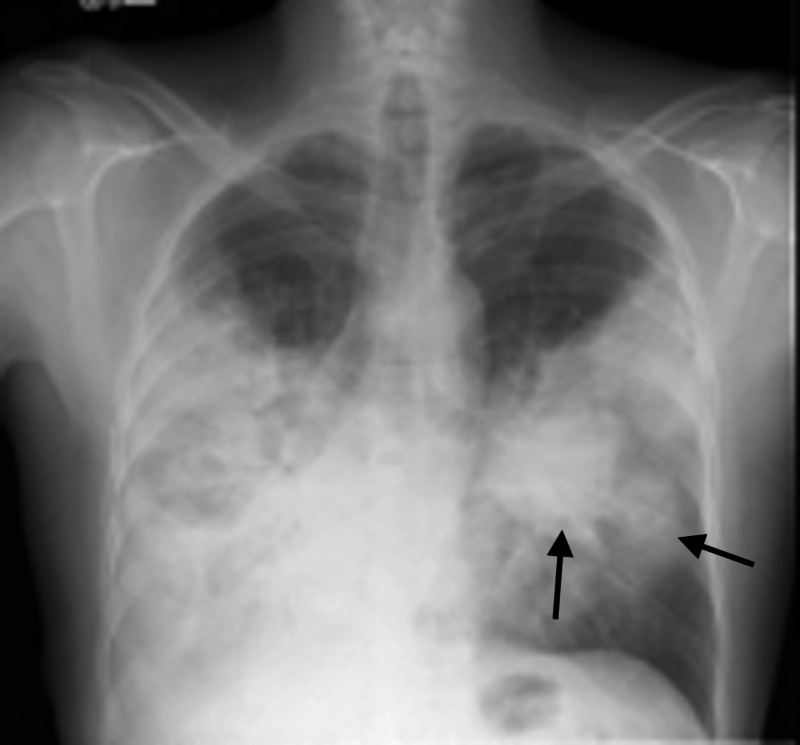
Chest X-ray three months prior to diagnosis Interval worsening of previously noted consolidations when compared to initial X-ray three weeks prior. Arrows identify nodules
